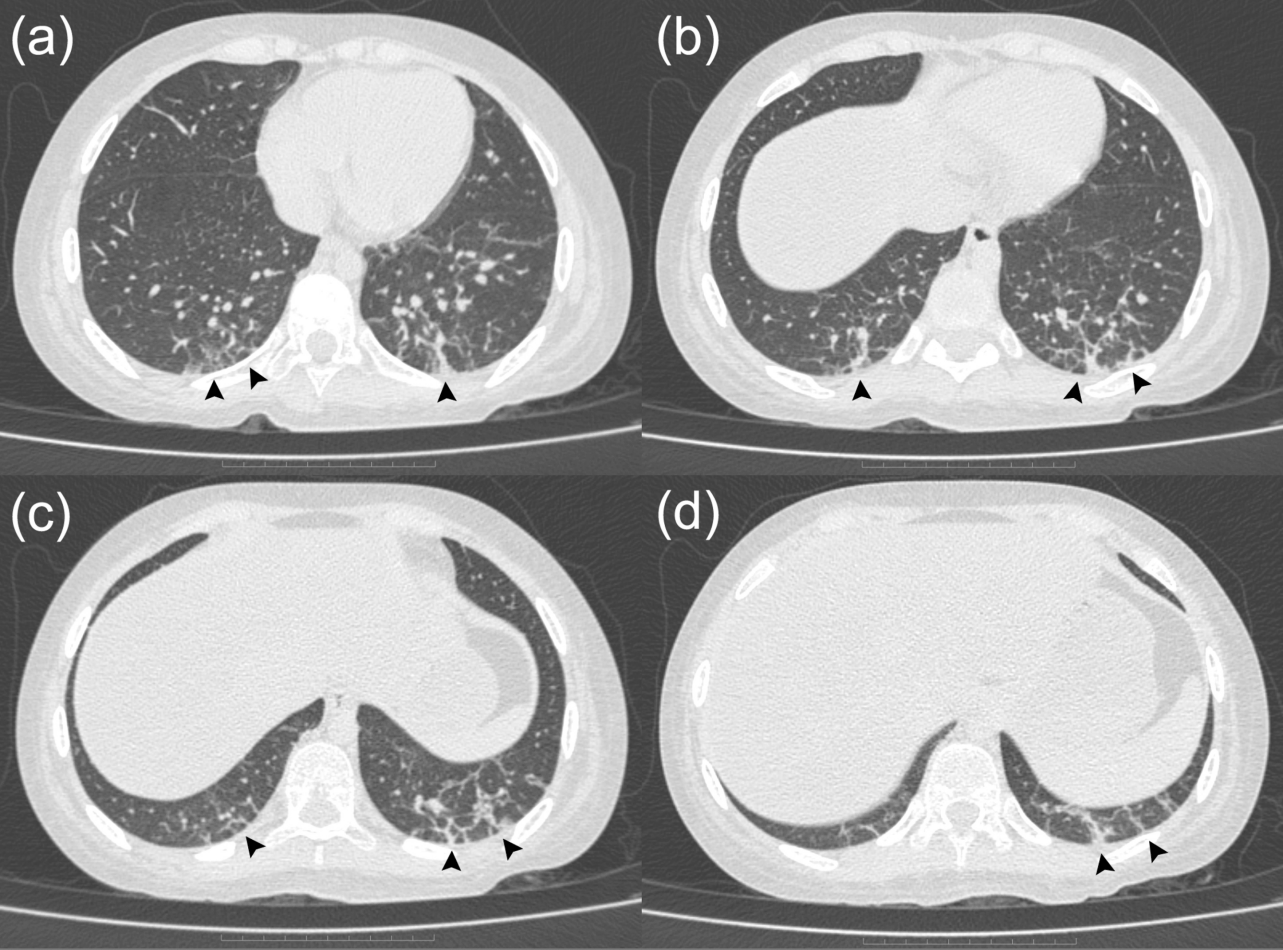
Axial non-contrast computed tomography of the lungs Black arrowheads indicate fibrosis: (a, b) middle pulmonary lobes; (c, d) lower pulmonary lobes
